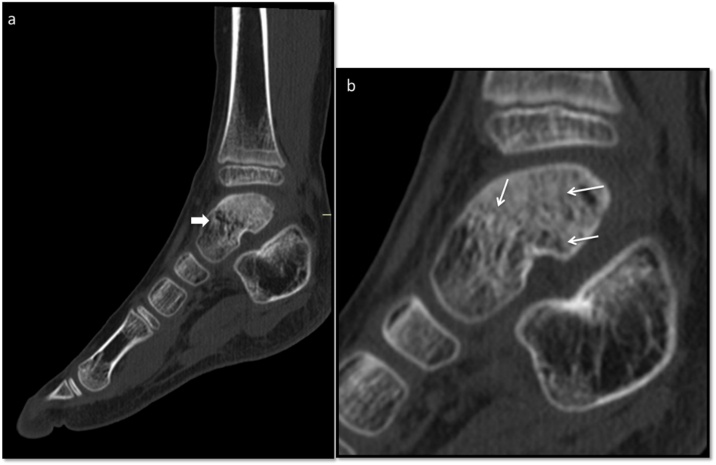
a and b. Six months after. CT scan, sagittal reconstruction with heterogenous traces of the talus body (arrow). Is evident the process of consolidation of the fracture (thin arrows).
